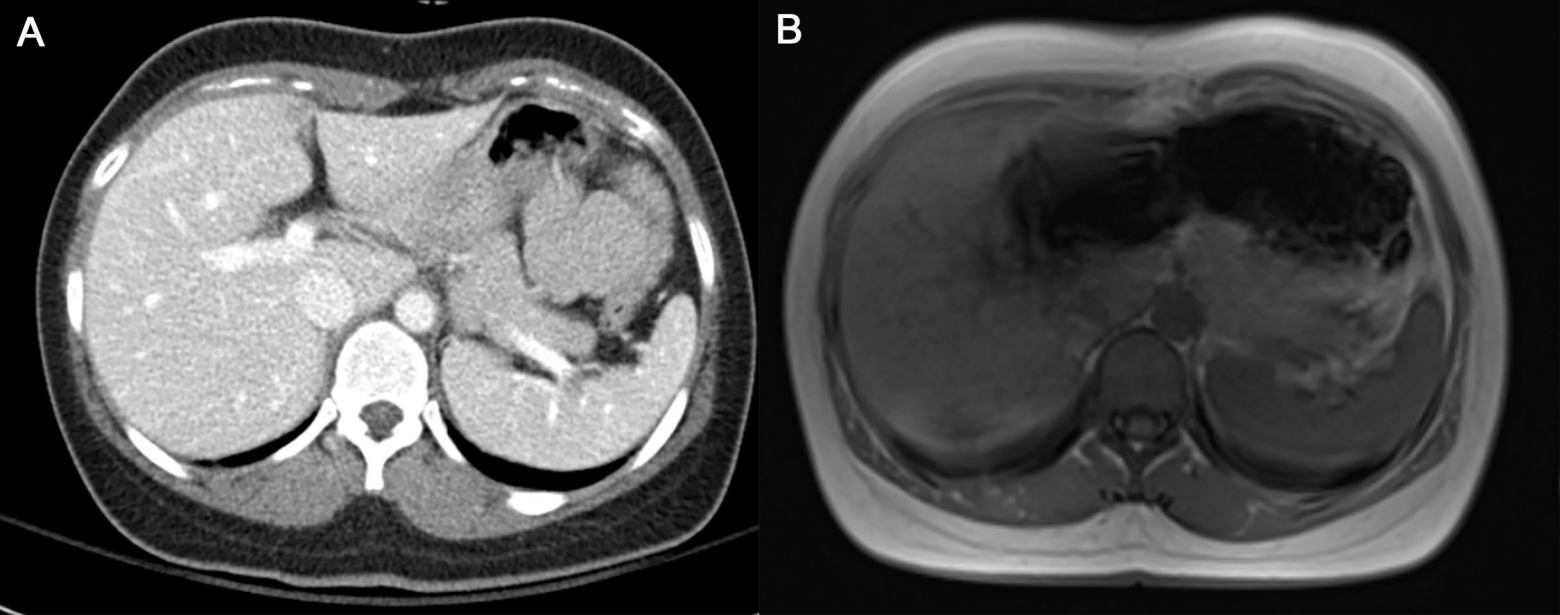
Quality of CT and MR images for TAMA measurement. (A) In the CT image, the abdominal muscle boundary is clear. (B) In the MR image, the presence of susceptibility and motion artifacts due to bowel gas degraded the image quality of the adjacent left rectus muscle and left psoas muscle.
